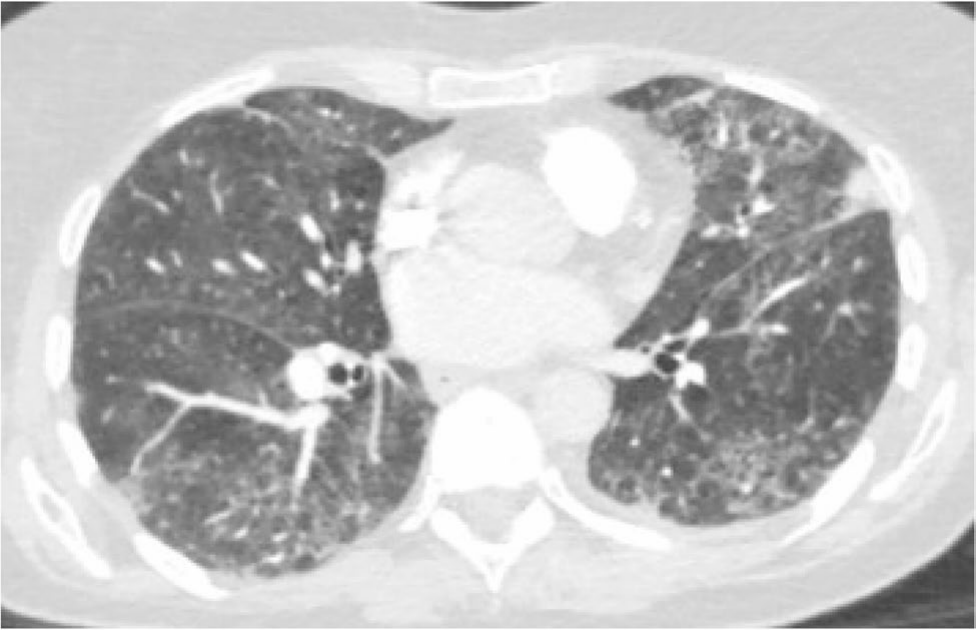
CT chest prior to treatment showing diffuse ground glass opacities
